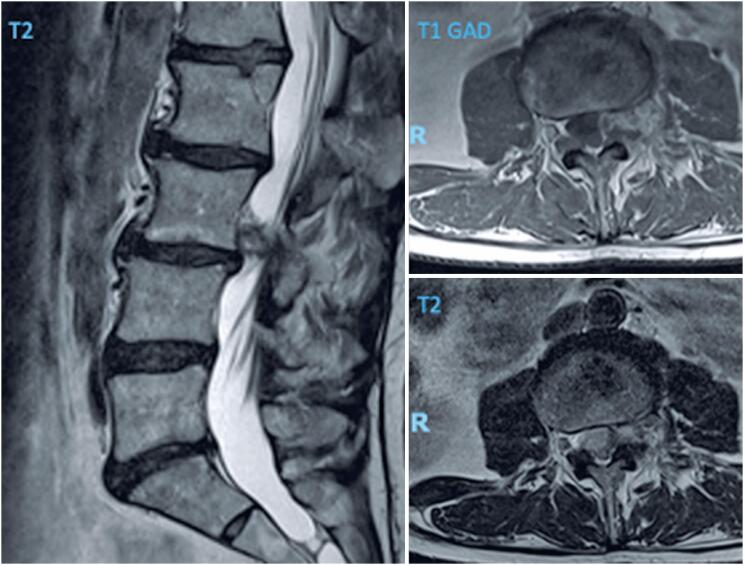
Sagittal central cut and axial images of the L3-L4 level from the post-procedure magnetic resonance imaging
